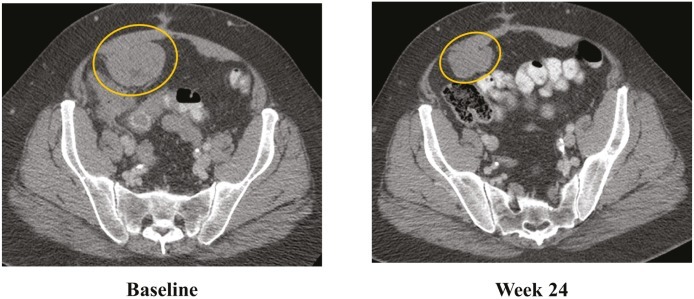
Tumor regression of 20% observed in abdominal and pelvic tumors on computerized tomography (CT) CT scan at (a) baseline and after (b) 24 weeks of treatment with BRAF inhibitor dabrafenib (GSK2118436).
